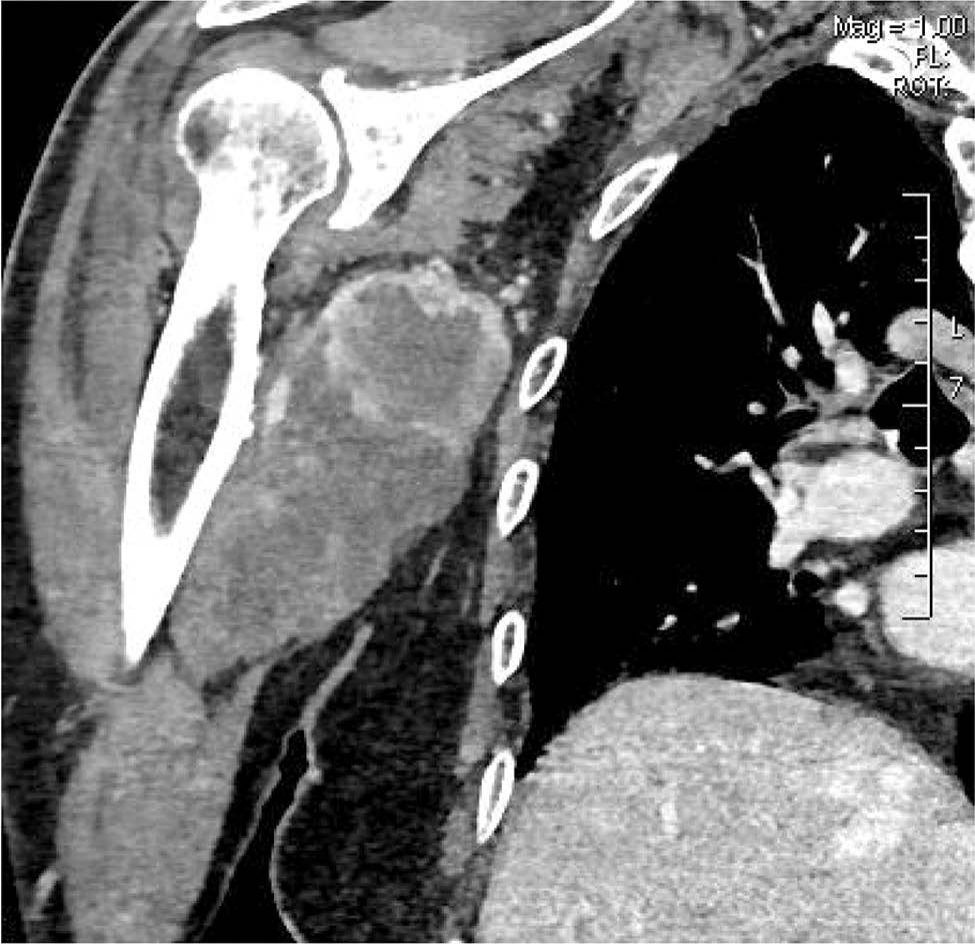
Contrast-enhanced computed tomography of the right axillary: a mixed density mass with rim enhancement.
